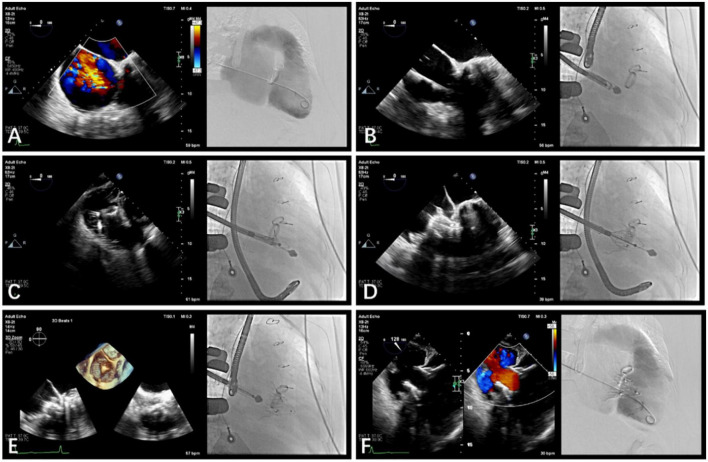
Guidance using transesophageal echocardiography (TEE) and fluoroscopic imaging in transcatheter tricuspid valve replacement. (A) TEE and fluoroscopy showed severe tricuspid re-gurgitation. (B) LuX-Valve guided by TEE was used to deliver the bioprosthesis to TA *via* the right intercostal approach. (C) The delivery system released the interventricular anchor and 2 graspers, and the graspers were guided by TEE to clamp the anterior leaflet. (D) The annulus skirt and the atrium disc were released in turn, and the position of the implant was adjusted by TEE to ensure that there was no obvious paravalvular leakage. (E) The bioprosthesis was completely re-leased after fixation with the interventricular anchor. (F) Postoperative computerized tomography angiography and TEE showed that tricuspid regurgitation disappeared immediately.
